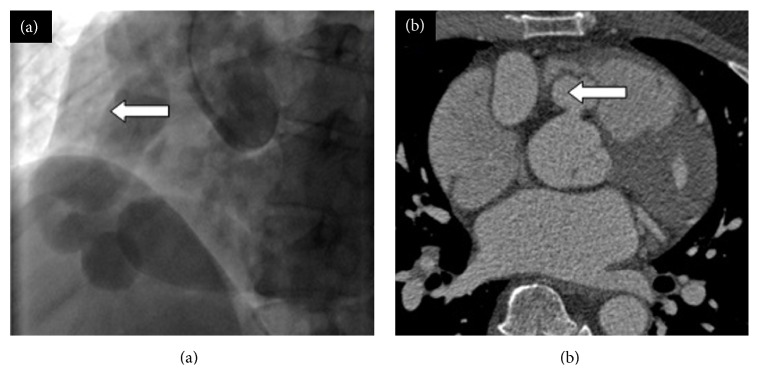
(a) Coronary angiogram demonstrating the aneurysmal right coronary artery on right coronary injection (arrow). (b) Computed tomography (CT) coronary angiogram showing the aneurysmal right coronary artery arising from the normal position on the aorta (arrow).
